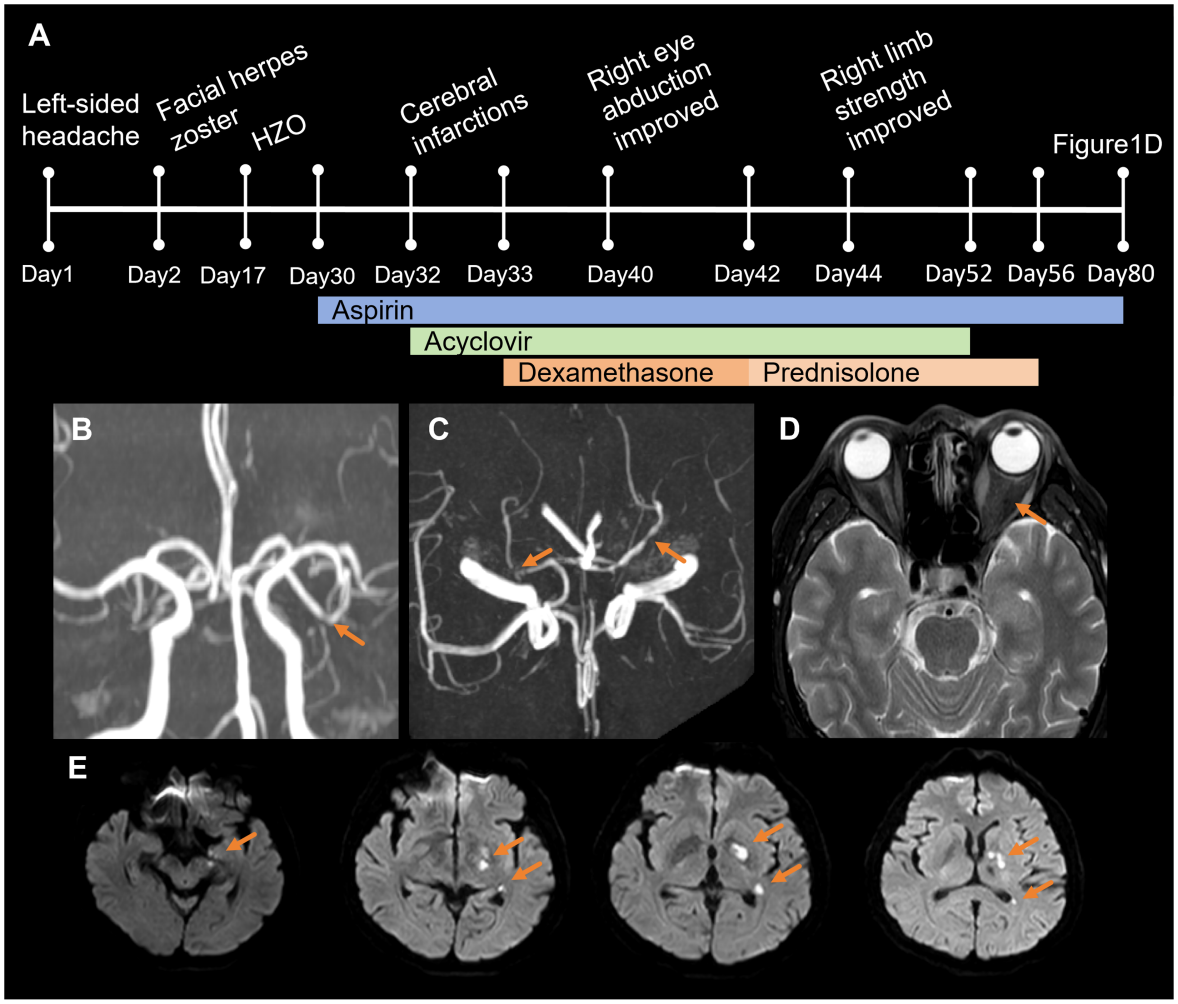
Timeline and magnetic resonance imaging. (A) Timeline summarizes the disease course and treatment. (B,C) Magnetic resonance angiography reveals that the lumen of the left middle cerebral artery is slightly narrowed in the M2 and M3 segments and limited slight stenosis in P1 segment of bilateral posterior cerebral arteries. (D) T2-hypointensity of the left optic nerve. (E) Diffusion-weighted imaging shows acute cerebral infarction in the left cerebral peduncle, parahippocampal gyrus, posterior limb of the internal capsule, globus pallidus, and paraventricular region.
